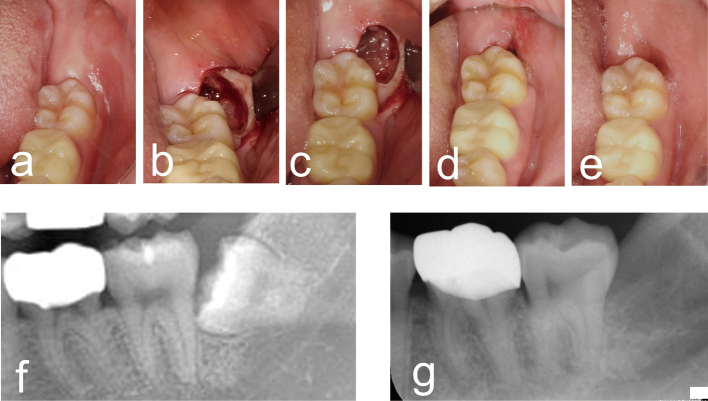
Clinical photographs and panoramic and dental X-ray images of PRP transplantation in a post-extraction socket case A 28-year-old female patient with a lower left wisdom tooth required extraction. Clinical photographs before tooth extraction (a), post-extraction (b), PRP transplantation (c), 1 week (d), and 4 weeks (e) after transplantation. Enlarged panoramic radiograph before tooth extraction (f) and dental radiograph 4 weeks after PRP transplantation (g).
